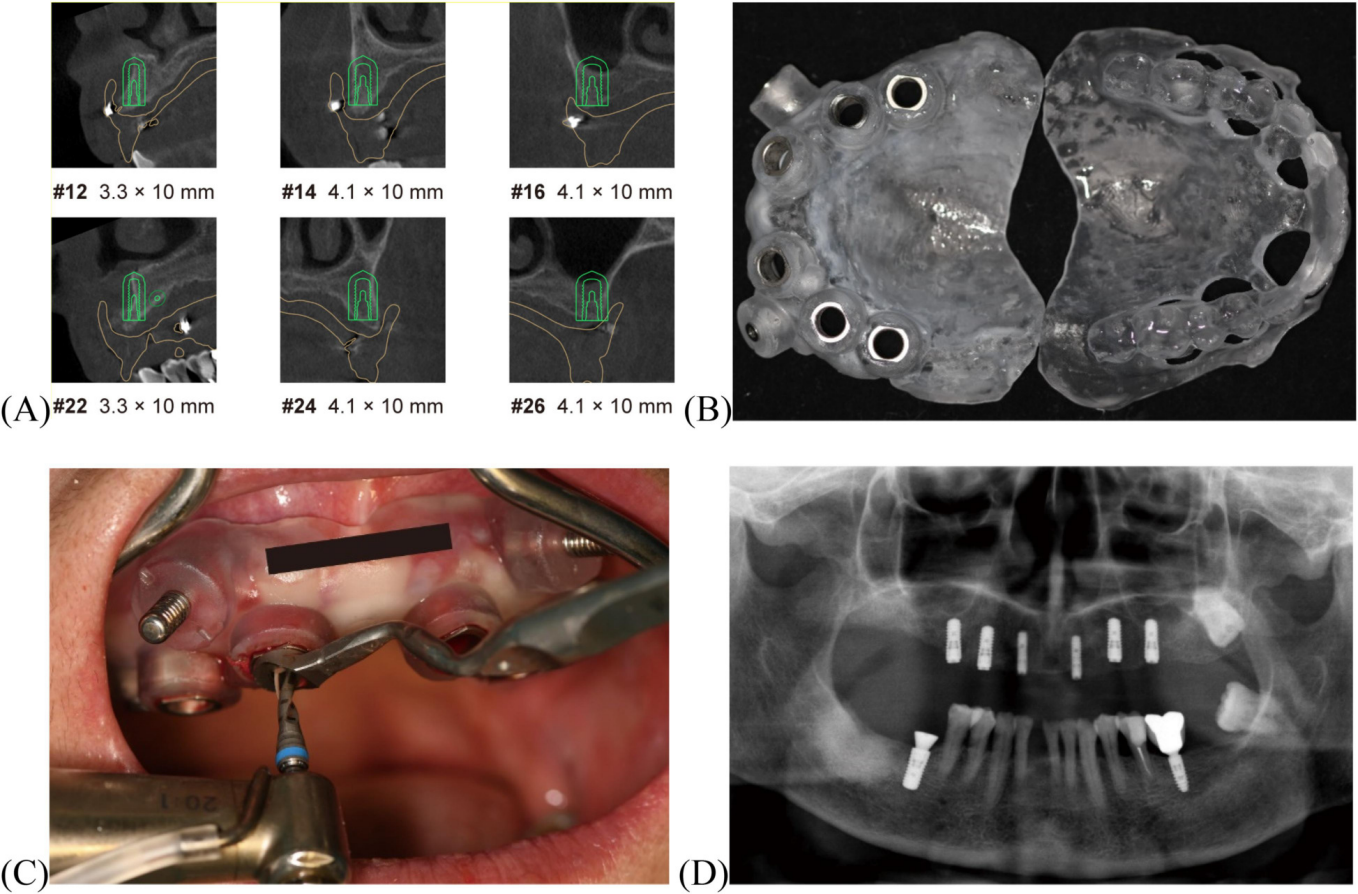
Digitally guided implant procedure. (A) CT image showing implant planning. (B) Implant surgical guide with occlusal registration. (C) Implant placement with guide. (D) Postoperative panoramic radiograph.
